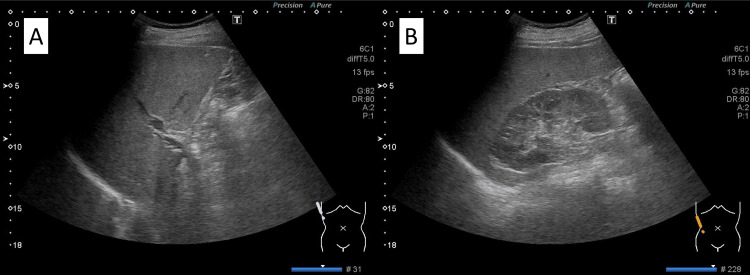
Abdominal ultrasonography of the liver Ultrasound examination of the abdomen showed increased echogenicity with a blunt liver edge. The gallbladder is absent, consistent with prior cholecystectomy.
